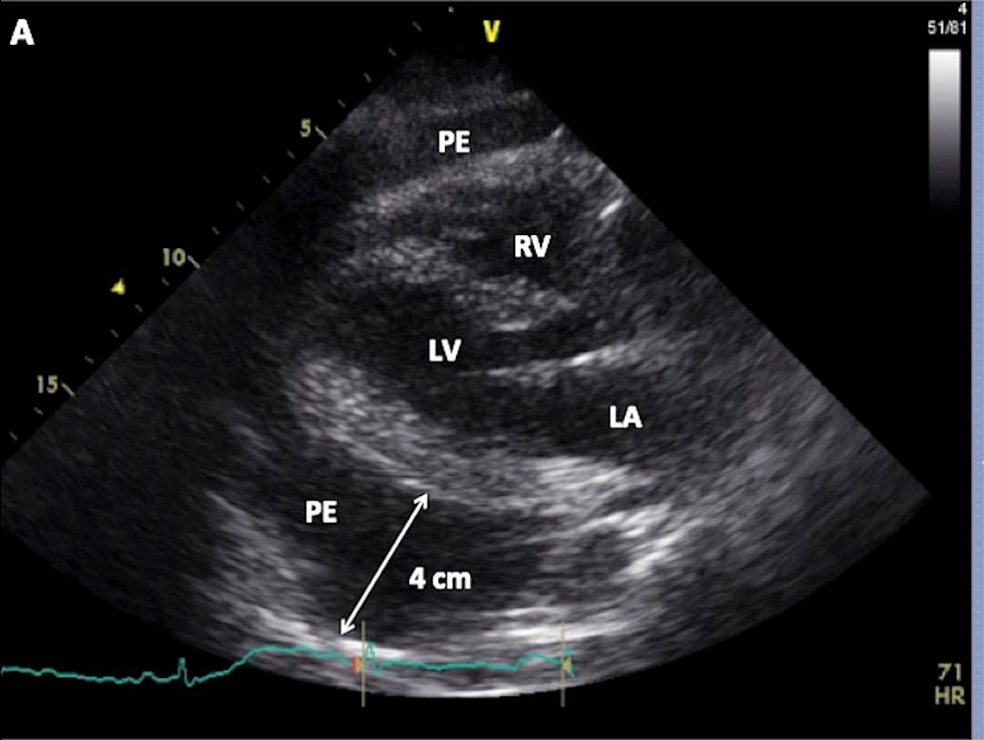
Echocardiographic image taken in the parasternal long axis view demonstrates a large pericardial effusion up to 4 cm PE: pericardial effusion; RV: right ventricle; LV: left ventricle; LA: left atrium.
